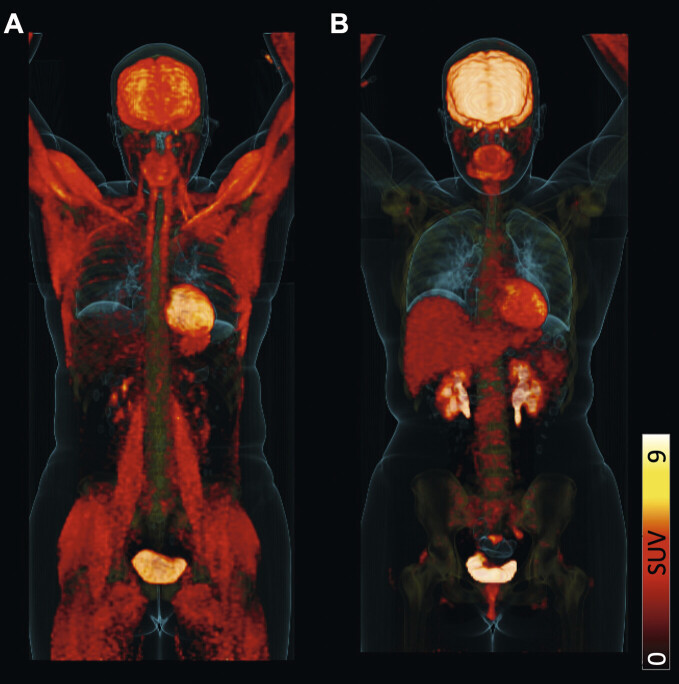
Patient with Hodgkin Lymphoma who was scheduled for [18F]FDG PET/CT. In (
